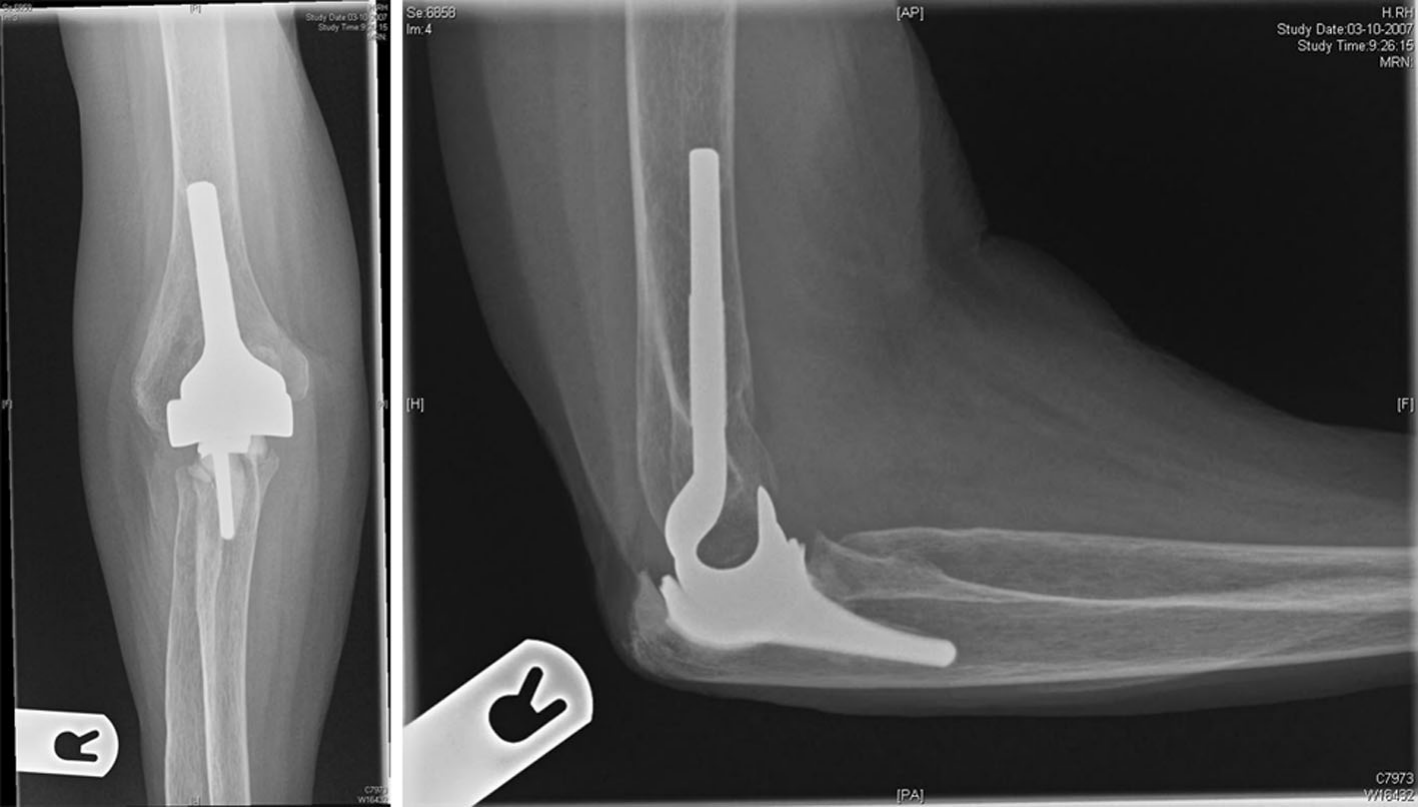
iBP Total Elbow System; there is no humeral anterior flange. This prosthesis has a characteristic hook-shaped humeral component, best seen on the lateral viewed radiograph. The radial component is relatively short
